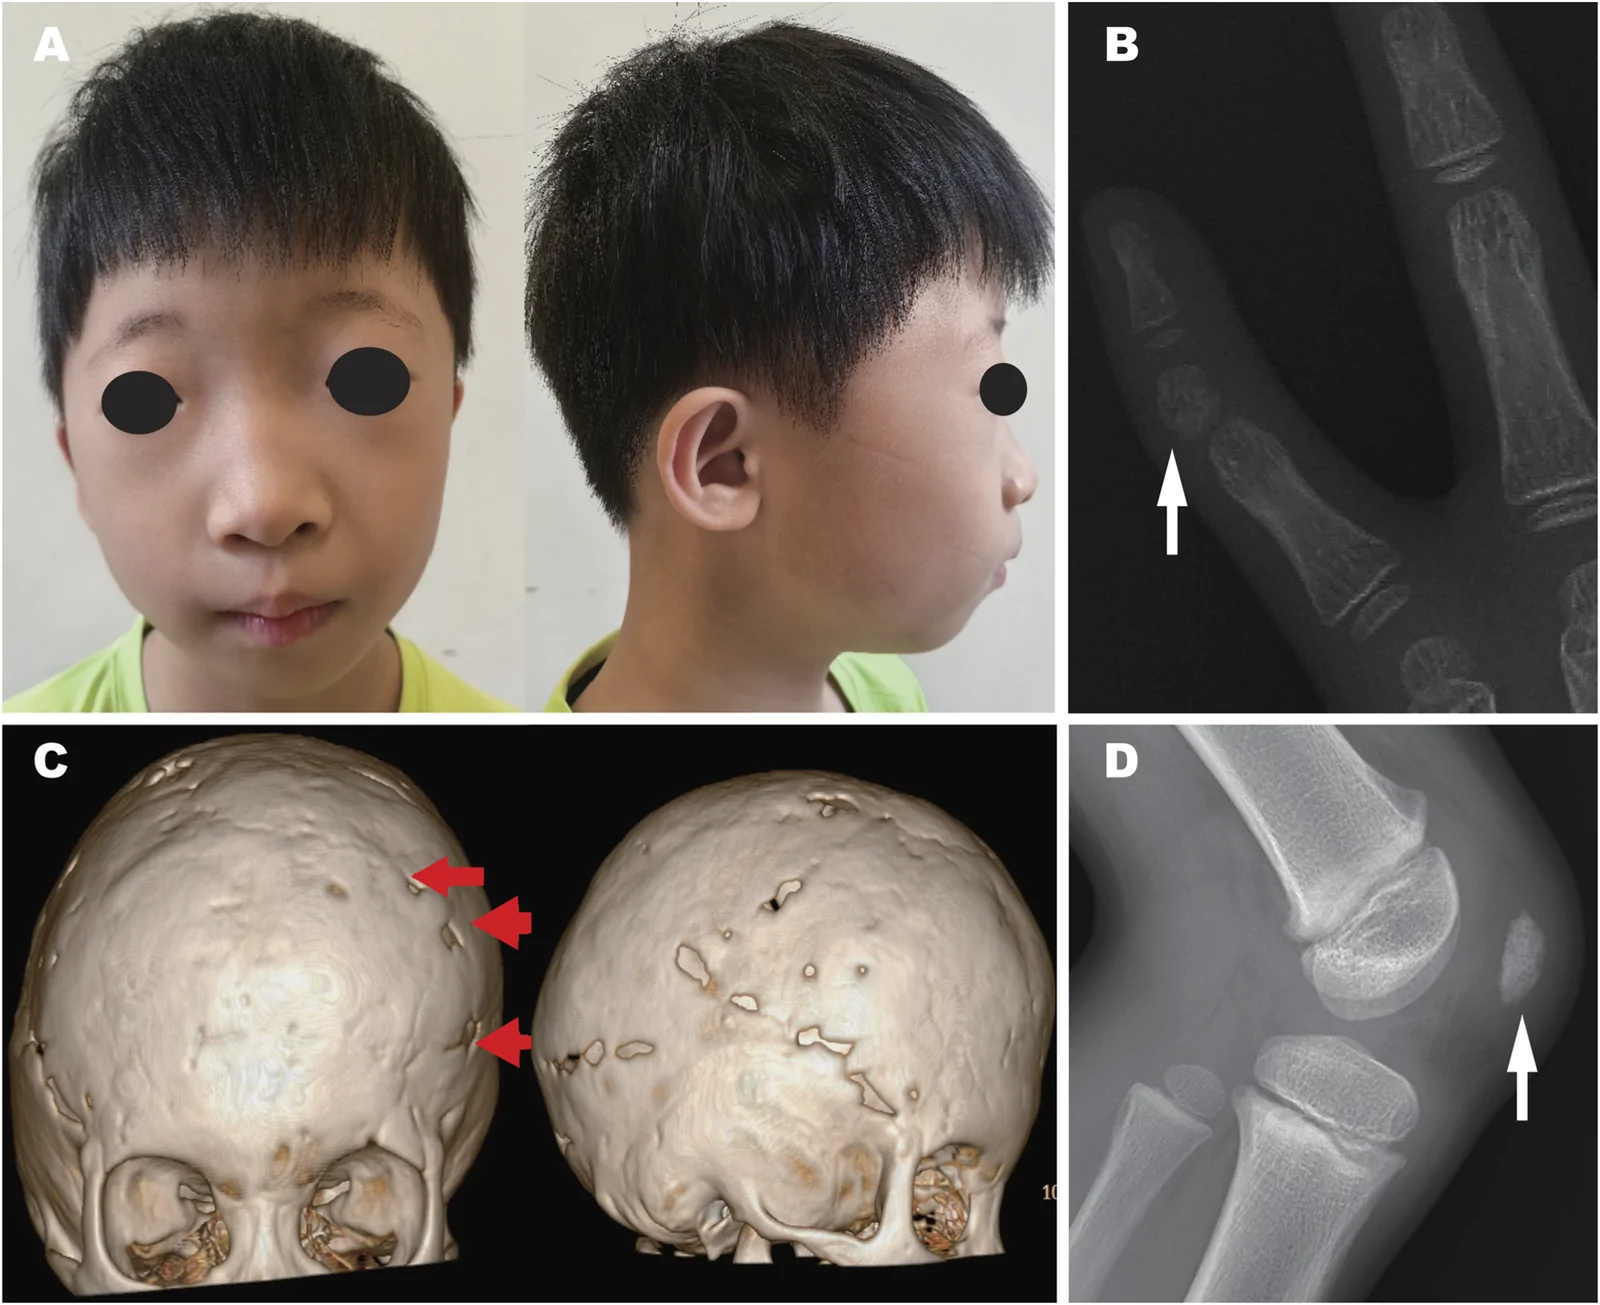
Skeletal abnormalities of the patient. (A) Craniofacial features. Supraorbital depression, minor mouth corner deviation, microstomia, prominent lips, and retrognathia. (B) Shortening of the left fifth middle phalanx (white arrows). (C) Cranial CT with VR reconstruction showing irregular skull contour with a widened forehead and elongated anteroposterior diameter. Multiple cranial sutures (frontal, sagittal, coronal, and lambdoid) and fontanelles are closed. Red arrows indicate multiple areas of irregular bony defects. (D) Patellar hypoplasia (white arrow).
